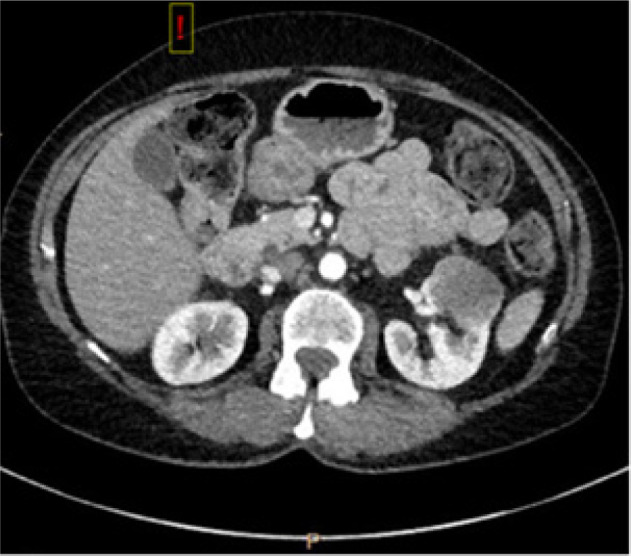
CT scan ordered by urgent care for presentation of shortness of breath with incidental finding of partially imaged heterogeneous, solid-appearing mass in the left kidney, approximately 3.4 × 2.6 cm.
